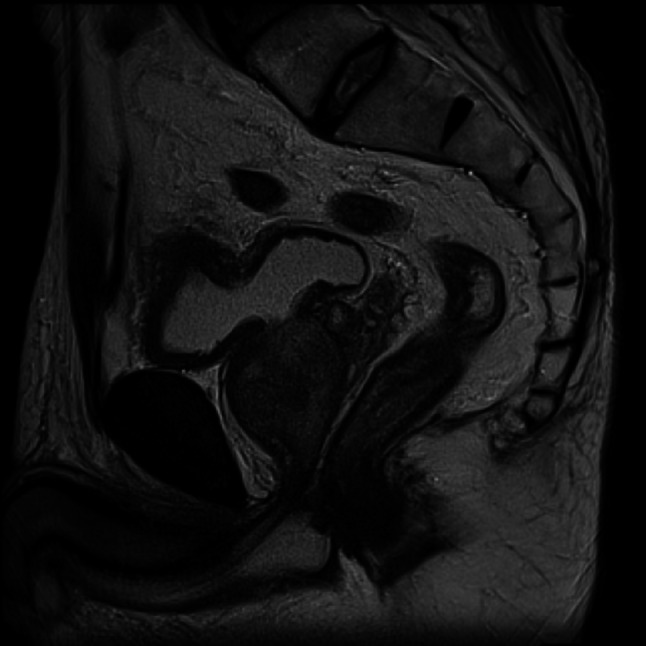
Contrast-enhanced MRI image of the bladder on presentation. Irregular thickening of the wall of the dome of the bladder showing gradual enhancement in a dynamic study. Restricted diffusion was observed
